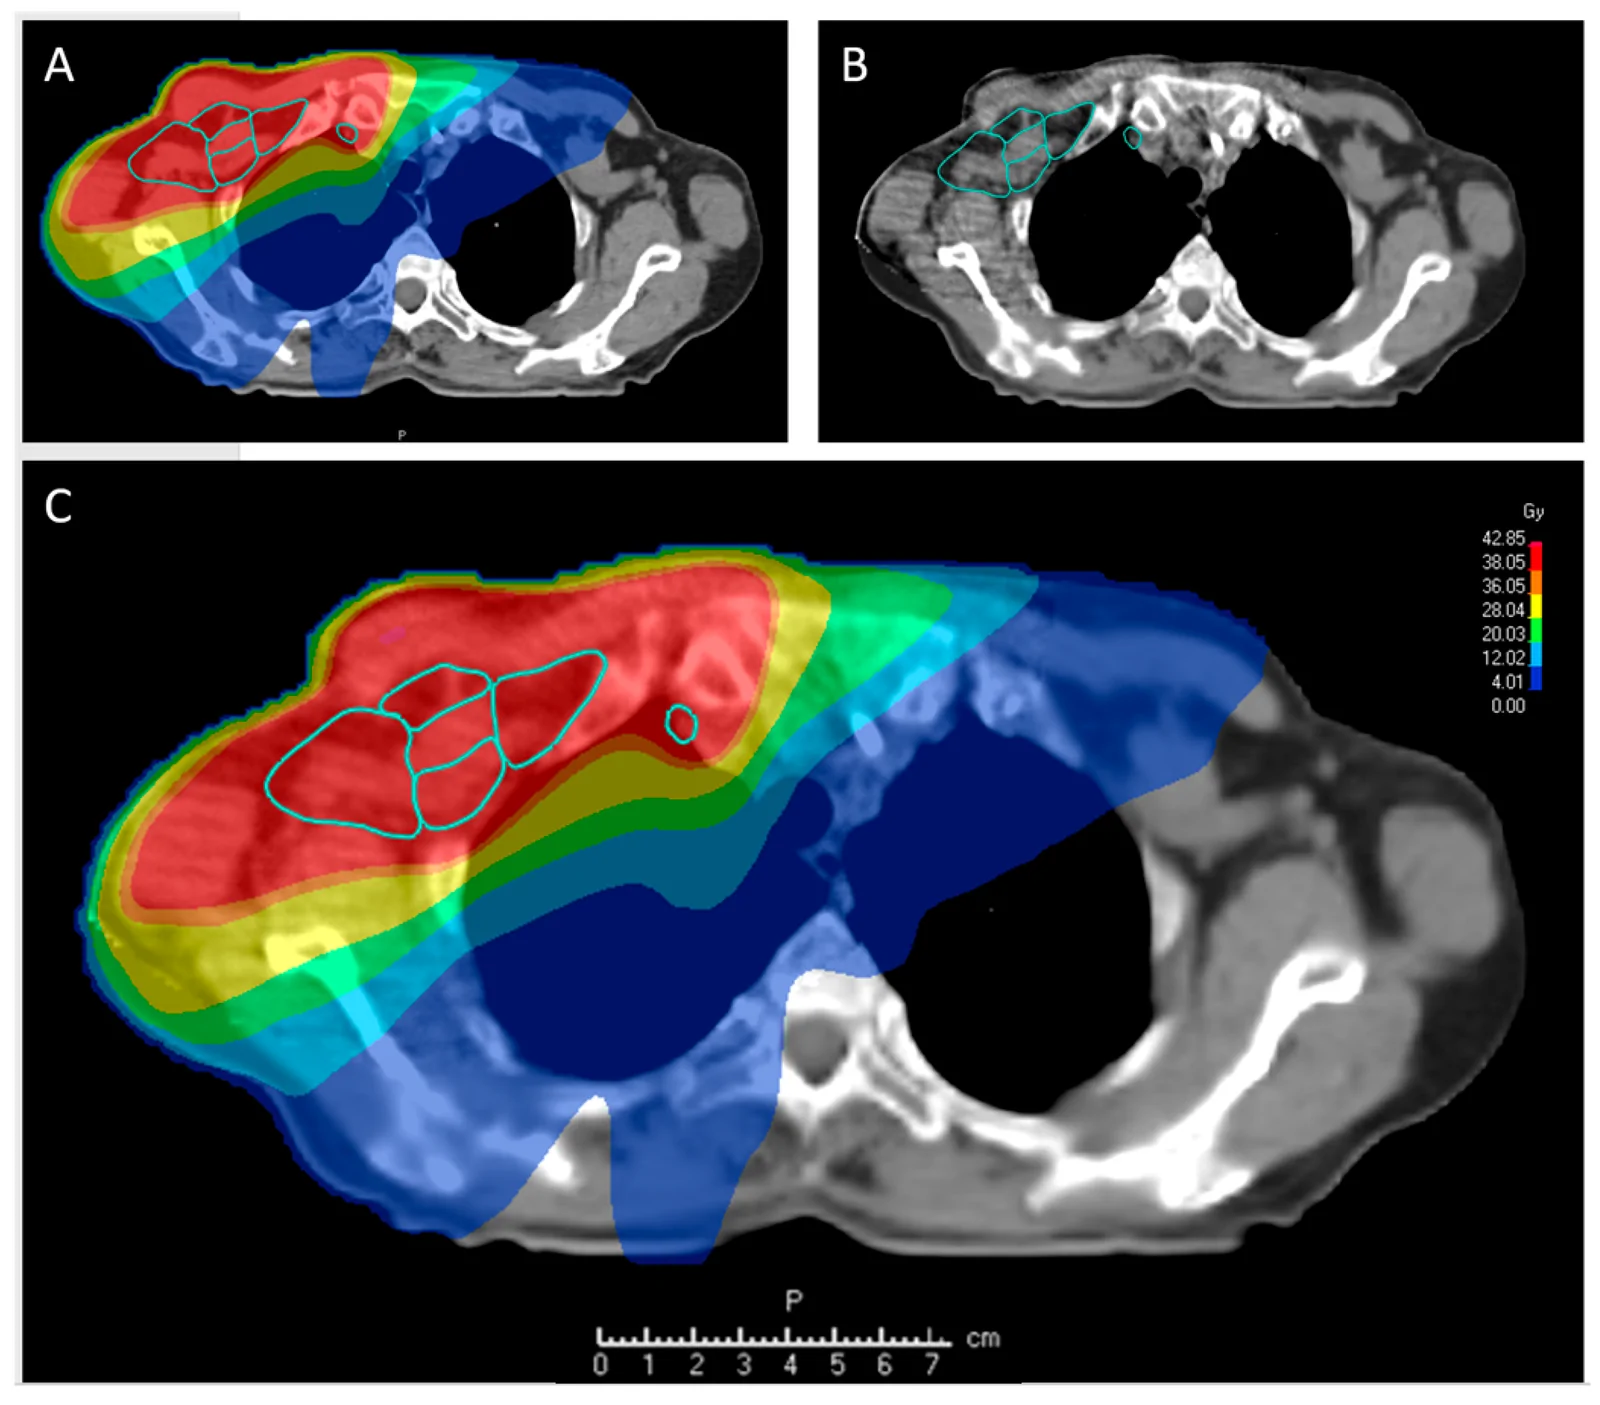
Forward calculation for a patient with right-sided regional nodal irradiation. Planning-CT and treatment plan (A), rigidly registered cone-beam computer tomography (B), and forward calculation (C).
